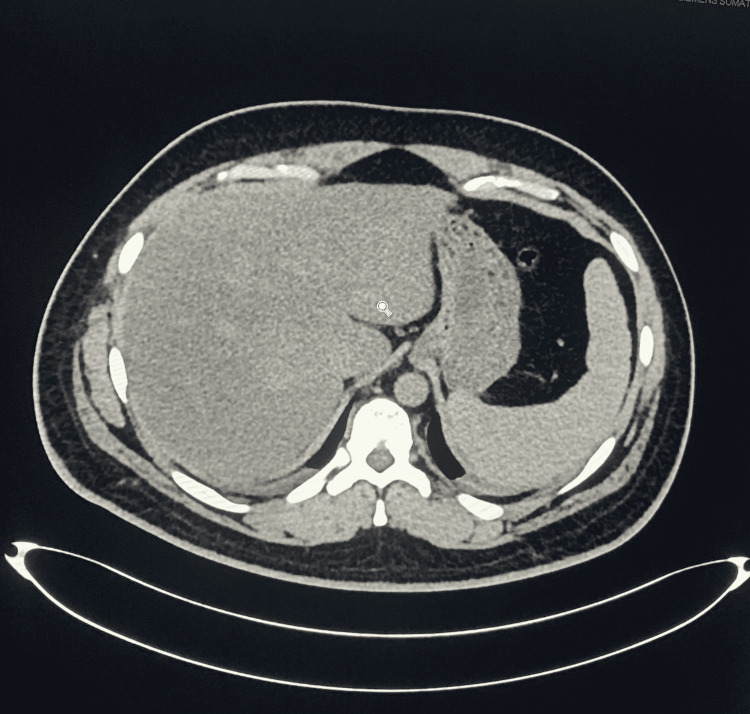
CT scan of abdomen (axial view without contrast).
